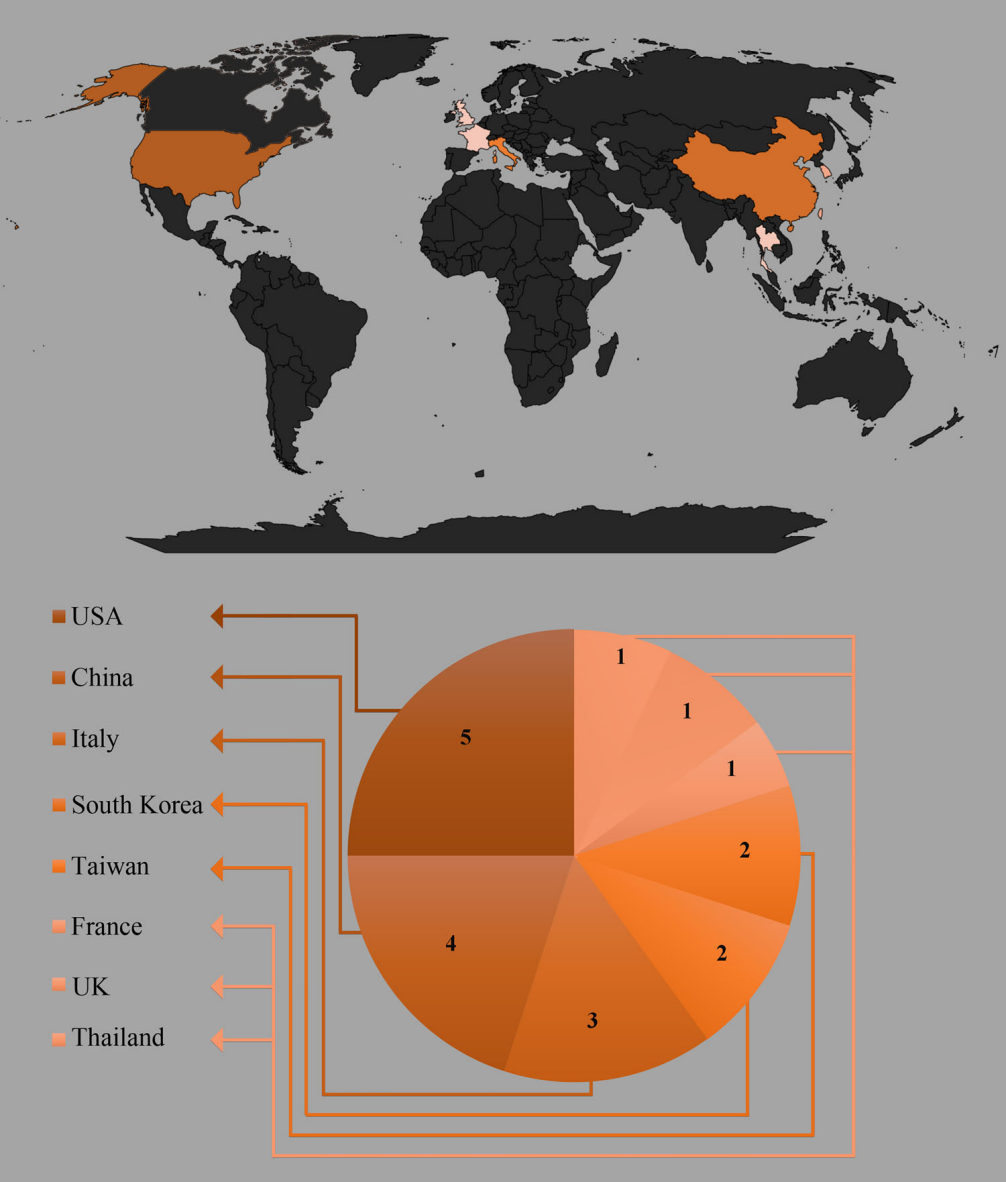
Geographic distribution of the countries and regions included in the systematic review of magnetic resonance imaging (MRI) biomarkers and neuropsychological assessments for obstructive sleep apnea (OSA) and attention deficits. The color gradient shows the proportion of articles from each country.
